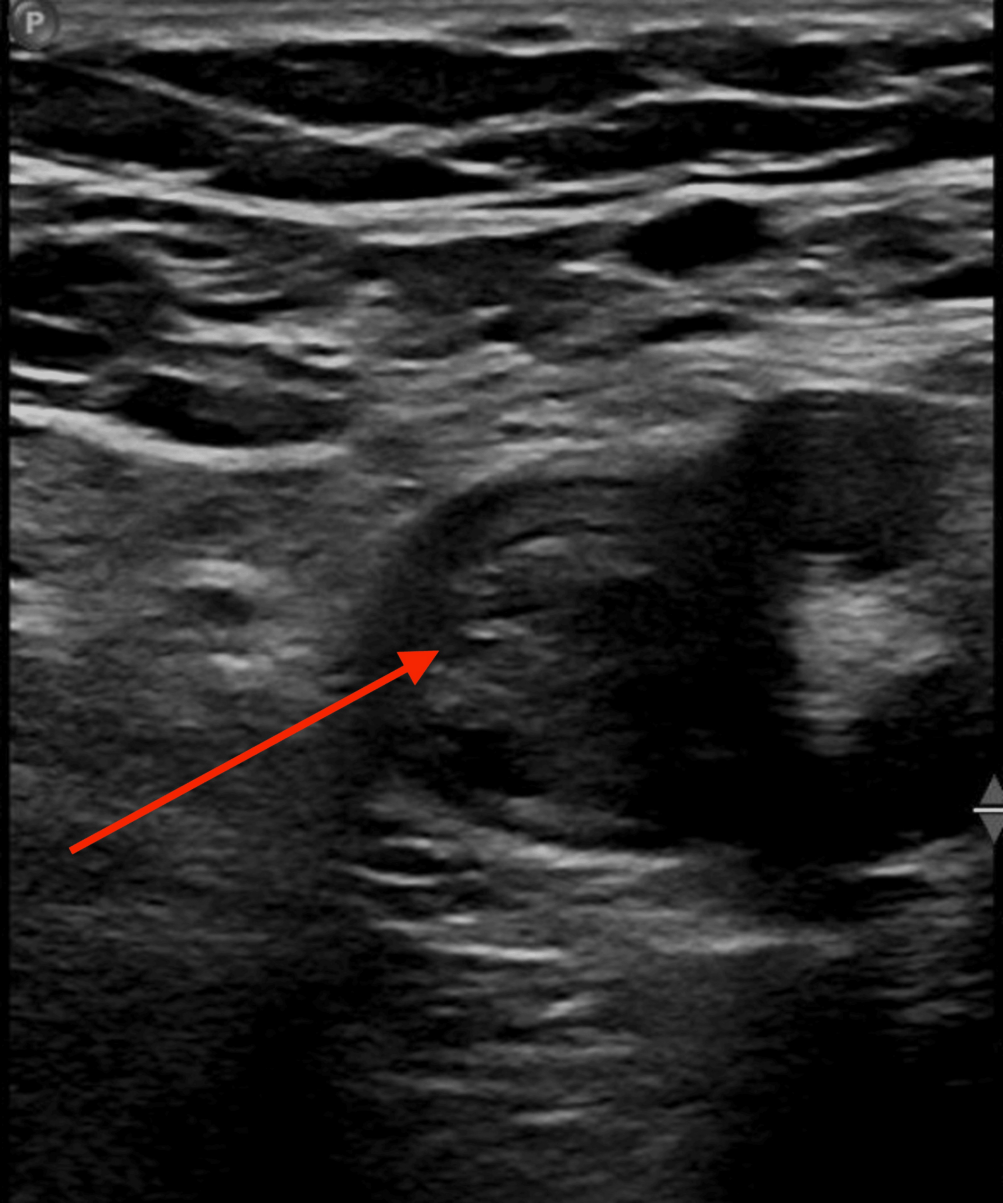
Ultrasound of the left common femoral vein demonstrating a large deep vein thrombosis Red Arrow: noncompressible thrombus in the left common femoral vein
